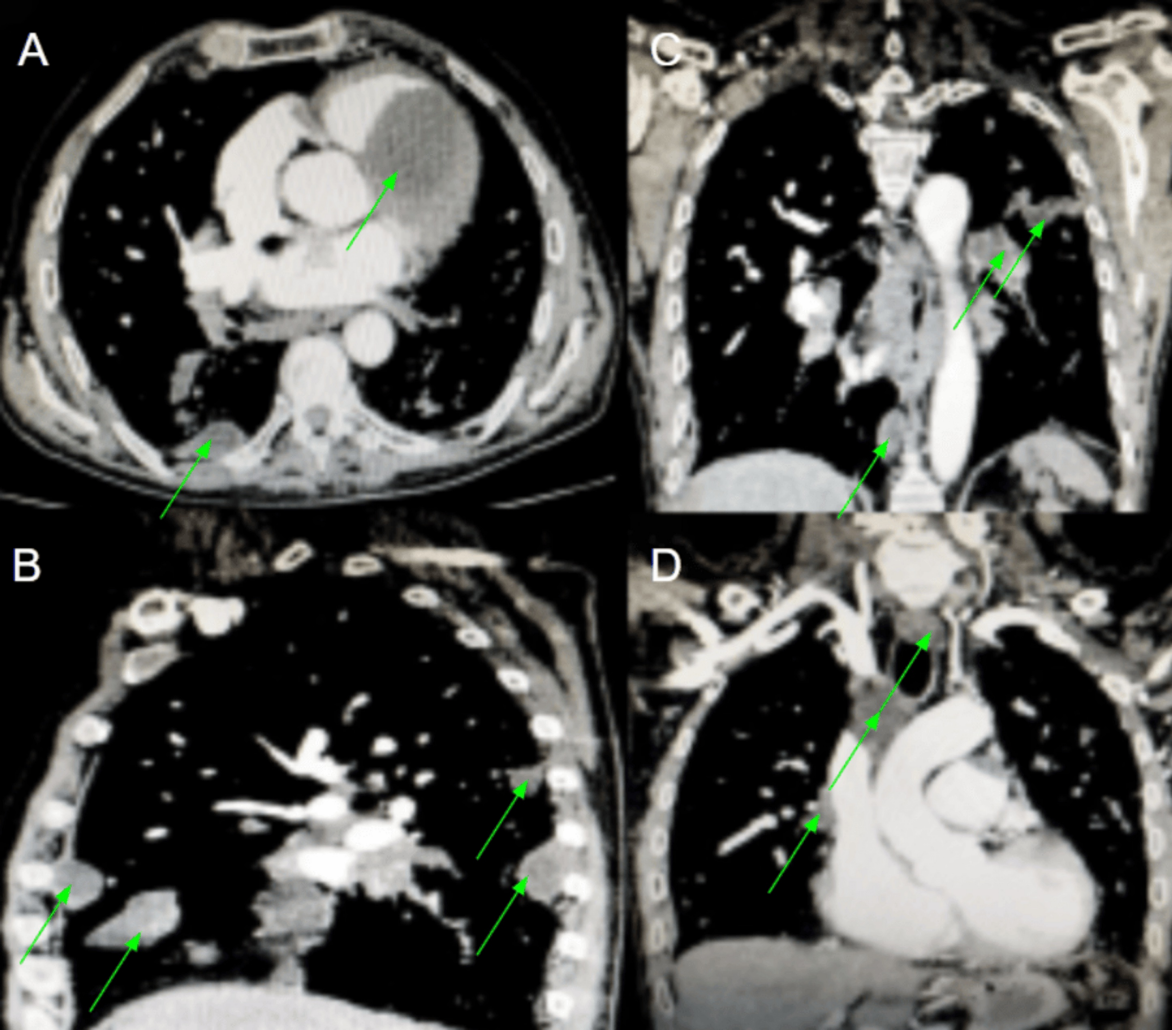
CECT images showing widespread mediastinal and pleuropulmonary hydatidosis including intracardiac, pericardial, and paravertebral hydatid cysts. Green arrow points to area of cysts. A. CECT chest axial image. B. CECT chest sagital reformatted image. C-D. CECT chest coronal reformatted images. CECT: Contrast-enhanced computed tomography
